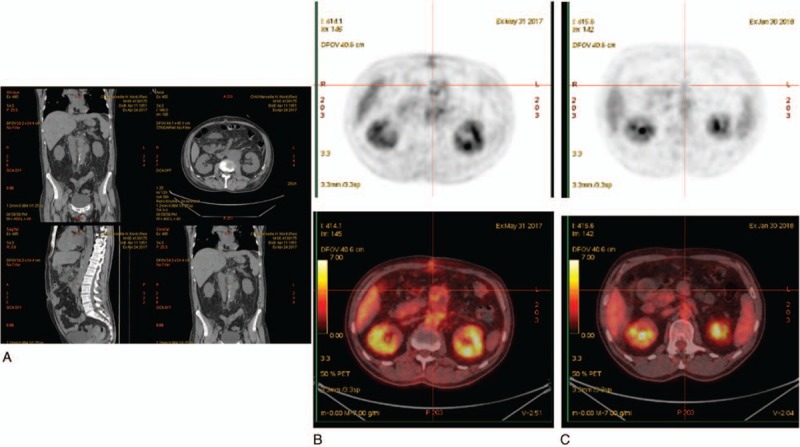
Thoraco-abdomino-pelvic computerized tomography scan showing sub-renal abdominal aorta pseudo-measuring 50 mm in the largest diameter with evidence of a collection in the left psoas muscle (A). Comparative 18FDG PET/CT before and after treatment: The 18FDG PET/CT performed before treatment showing intense hypermetabolism of the aneurysm (B) and the 18FDG PET/CT performed after treatment, showing reduction of the metabolism (C). 18FDG PET/CT = 2-deoxy-2-[fluorine-18]fluoro-D-glucose positron emission tomography combined with computed tomography.
